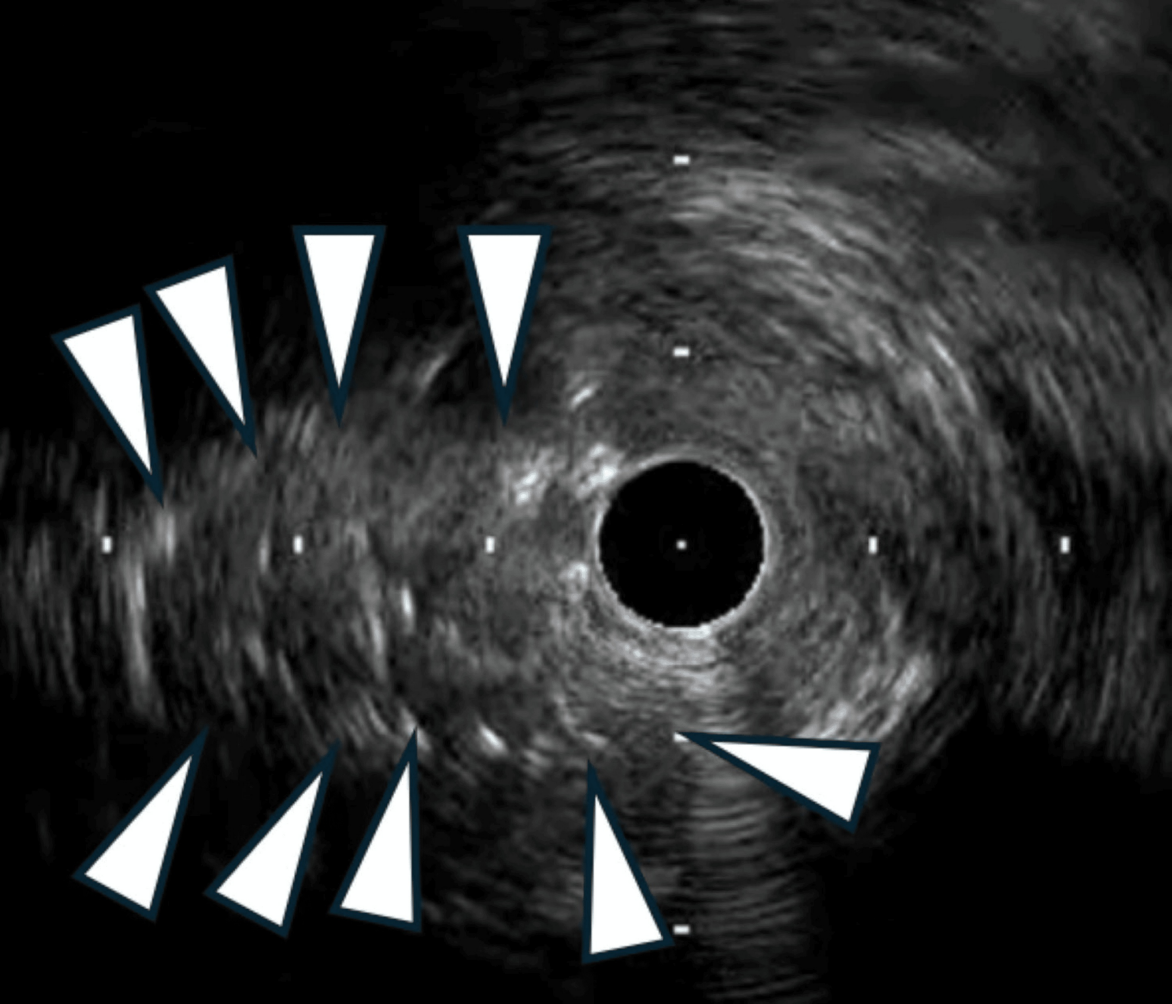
Intravascular ultrasound confirming the guidewire advanced outside the stent nodule (white arrowhead)
